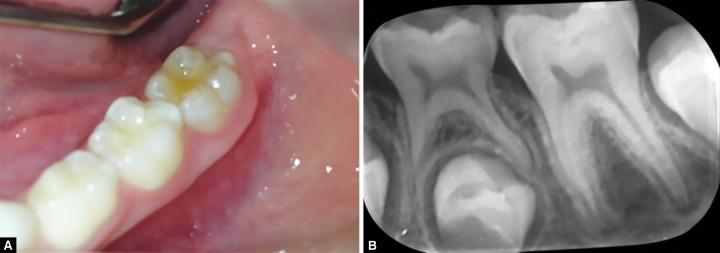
(A) Clinical aspect after 18 months of follow-up since the beginning of clinical approach. (B) Radiograph image of the 18-month follow-up
